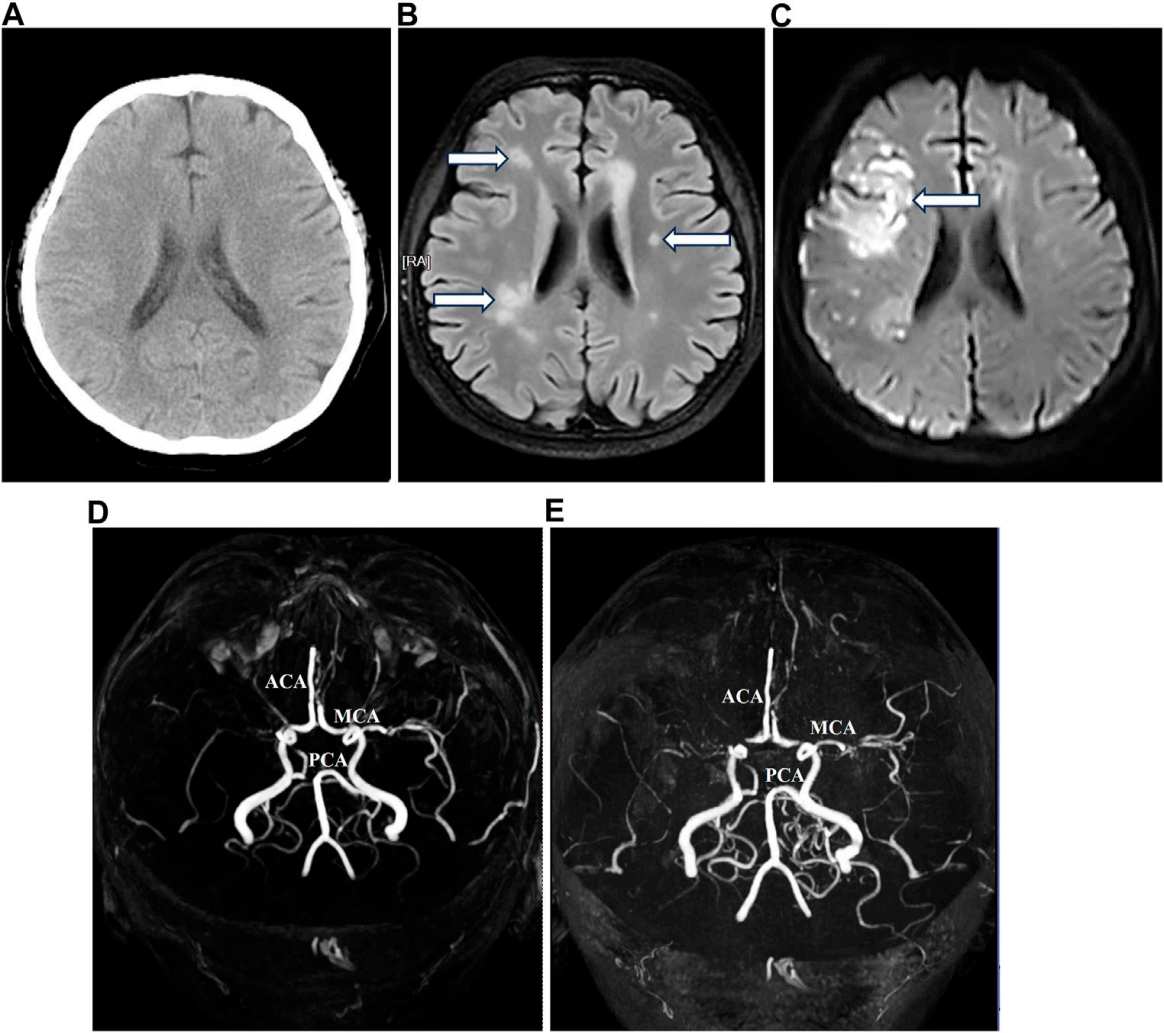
Magnetic resonance imaging and magnetic resonance angiography before and after chemotherapy.
